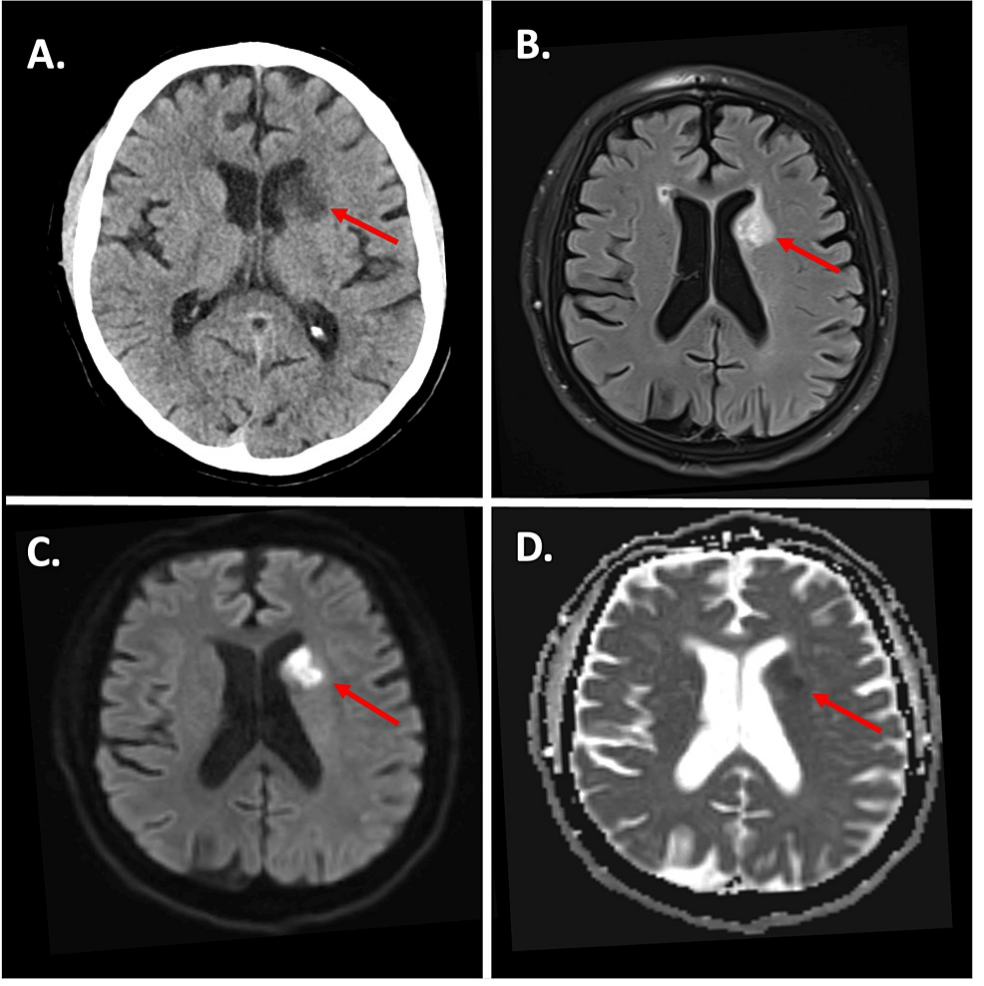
CT and MRI following left caudate nucleus stroke A. CT head showing hypodensity in the left basal ganglia; B. FLAIR sequence of MRI showing hyperintensity in the left caudate nucleus; C and D. DWI and ADC sequences of MRI showing restricted diffusion in the left caudate nucleus (red arrows). FLAIR: fluid-attenuated inversion recovery; DWI: diffusion-weighted imaging; ADC: apparent diffusion coefficient
